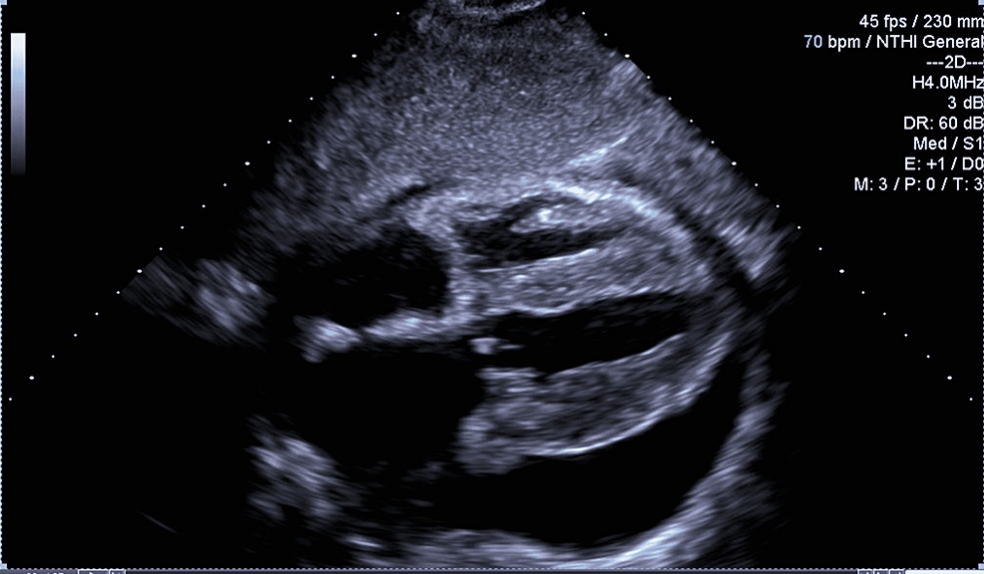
A bedside echocardiogram revealed massive pericardial effusion.
